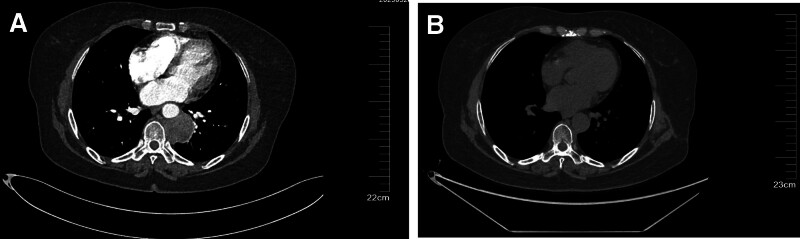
Imaging manifestations of patient. (A) CT manifestations paraspinal space in the lower left lung, which was closely related to the thoracic aorta. (B) CT manifestations of review. CT = computed tomography.
